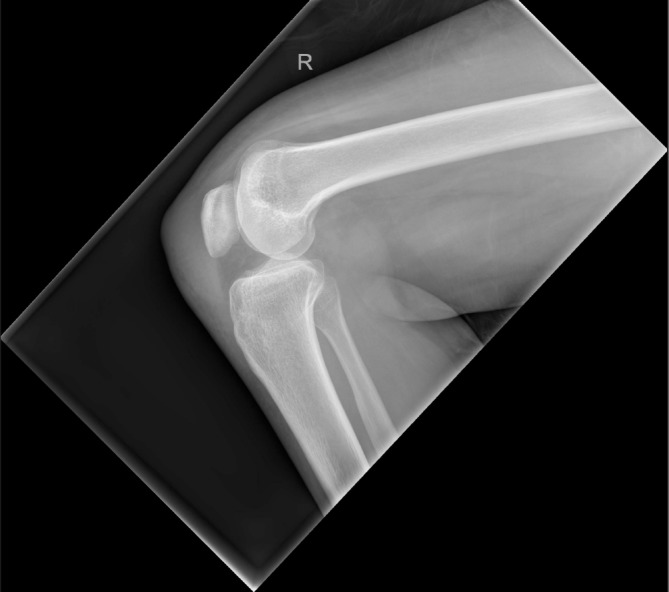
Plain lateral x-ray of the knee showing prepatellar soft tissue swelling; no obvious bony lesion
